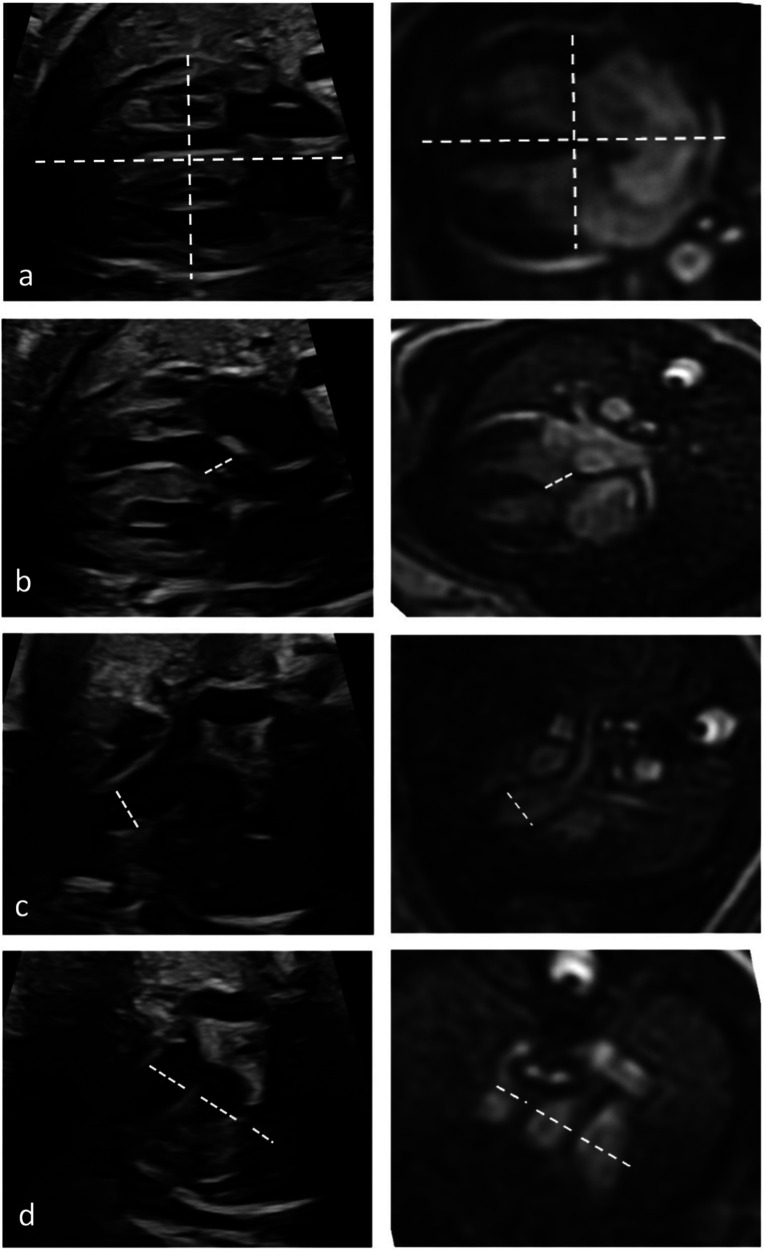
Quantitative assessment of cardiac diameters. Diameter measurements in fetal cardiac five axial views in a healthy Fetus (35 + 1 weeks gestation) in fetal echocardiography (left column) and cardiac MRI (right column). Longitudinal and transverse cardiac diameter from 4-chmaber view (a), diameter of the LVOT (b), the RVOT diameter (c) and diameters of pulmonary artery, aorta, and superior vena cava from the three-vessel view (d).
